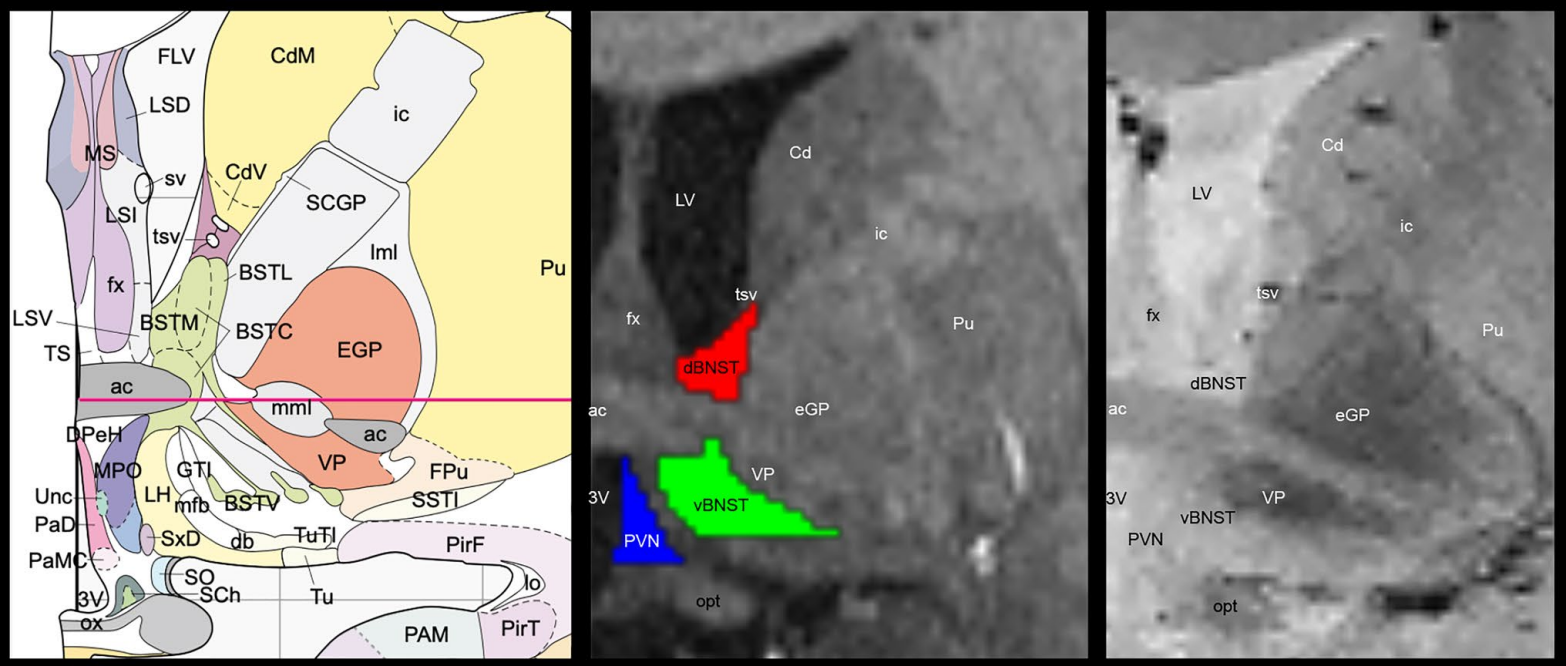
The paraventricular nucleus of the hypothalamus (PVN) and the bed nucleus of the stria terminalis (BNST) and their surrounding boundary-setting structures; tract. Left = Atlas of the Human Brain (Mai et al. 2008; ‘BST’ structures used to depict dorsal and ventral BNST and PaD (paraventricular nucleus, dorsal part) and PaMC (paraventricular nucleus, magnocellular part) used to depict PVN structure); Middle = T1-weighted MPRAGE with segmentations (dorsal BNST, red; ventral BNST, green; PVN, blue); Right = high-resolution gradient echo (2D GRE). *dBNST* dorsal bed nucleus of stria terminalis, *vBNST* ventral bed nucleus of stria terminalis, *PVN* paraventricular nucleus of the hypothalamus, *ac* anterior commissure, *Cd* caudate, *eGP* external globus pallidus, *ic* internal capsule, *LV* lateral ventricles, *tsv* thalamostriate vein, *VP* ventral pallidum, *Pu* putamen, *3V* third ventricle, *opt* optic tract
